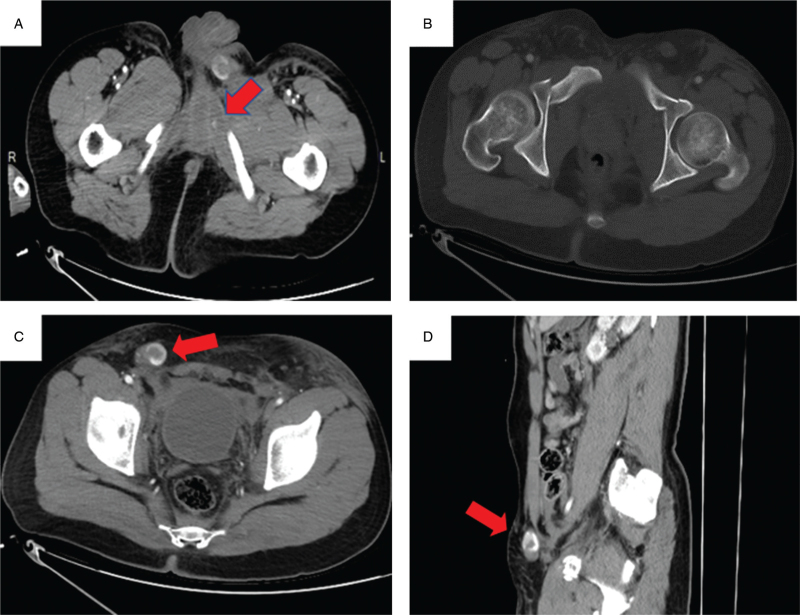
A: CT imaging showing bleeding in left levator ani. B: CT imaging showing bilateral pubic superior and inferior ramus fractures. C: CT imaging showing right testicular dislocation(axial view). D: CT imaging showing right testicular dislocation (sagittal view). CT = computed tomography.
